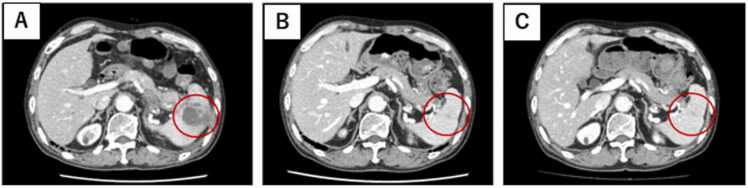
Abdominal computed tomography (CT) shows that anti-tuberculous treatment reduced the size of the lesion. A) CT on admission, B) CT at 5 months of treatment, C) CT at 9 months after the completion of treatment.
